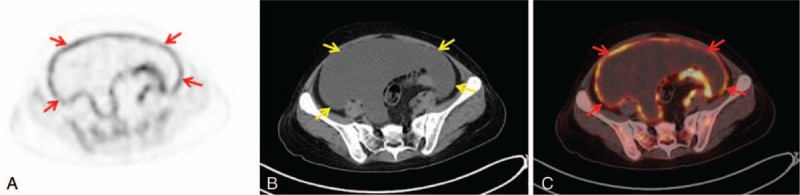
A 37-year-old man with tuberculous peritonitis: string-of-beads ^18^F-FDG uptake in the parietal peritoneum (red arrow) on PET and combined PET/CT axial imaging (A and C); smooth uniform thickening (yellow arrow) on axial CT imaging (B). CT = computed tomography,^ 18^F-FDG = F18-fluorodeoxyglucose, PET = positron emission tomography.
